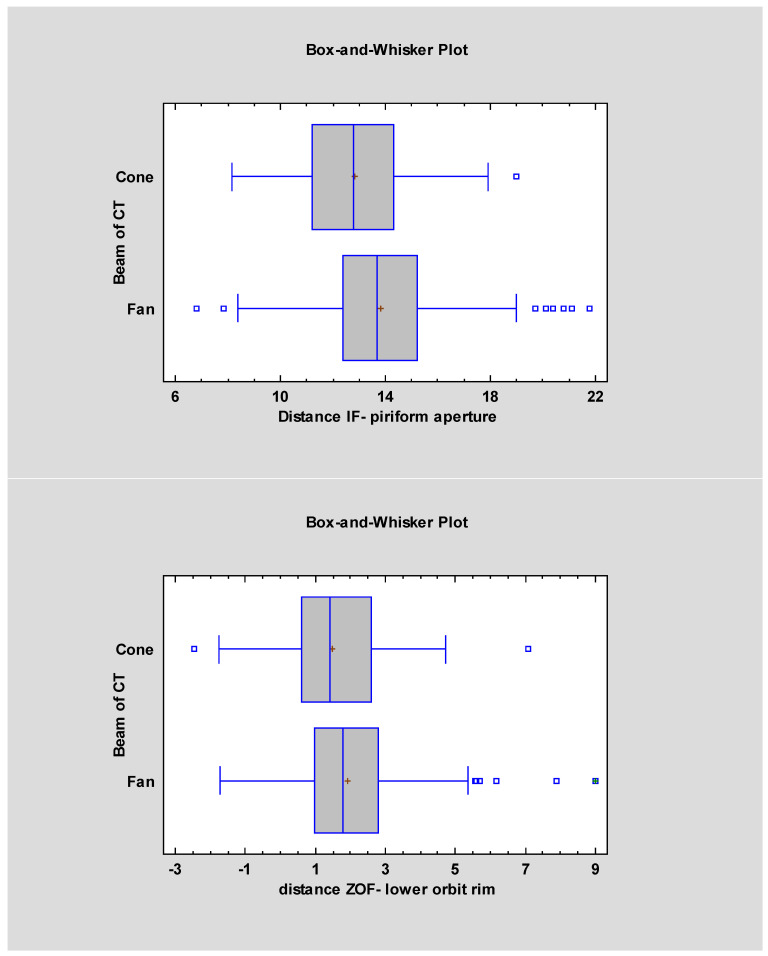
Measurements showing statistically significant differences (*p* < 0.05) between models segmented from cone-beam and fan-beam CT data.
